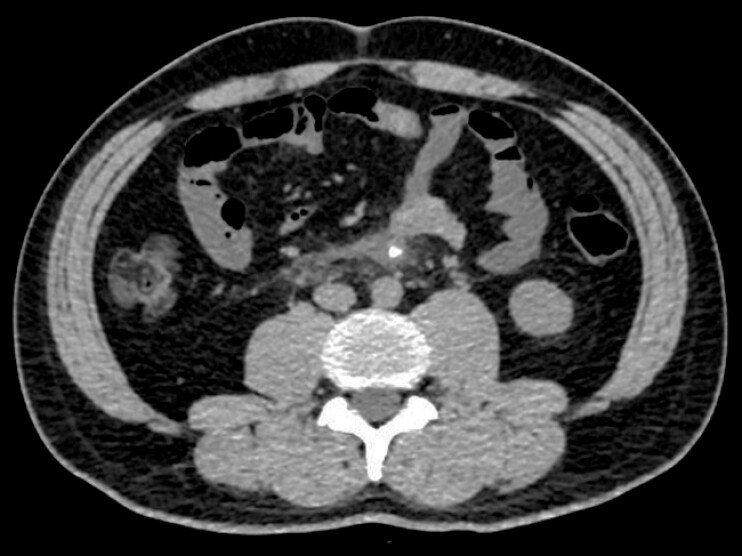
Abdominal CT showed that one of the wires was likely embedded in the wall of the horizontal duodenum, causing localized inflammatory exudate without evidence of perforation. CT, computed tomography.
